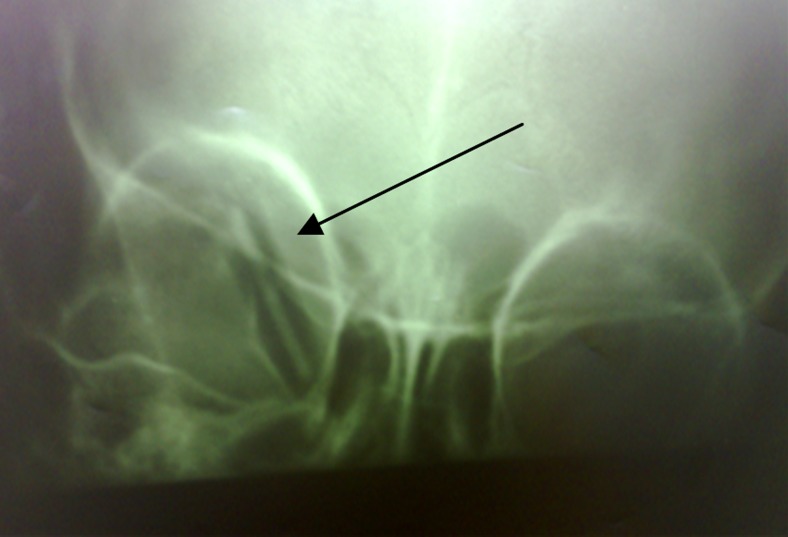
X-ray of the existence of an object with low density in retro-orbital space
